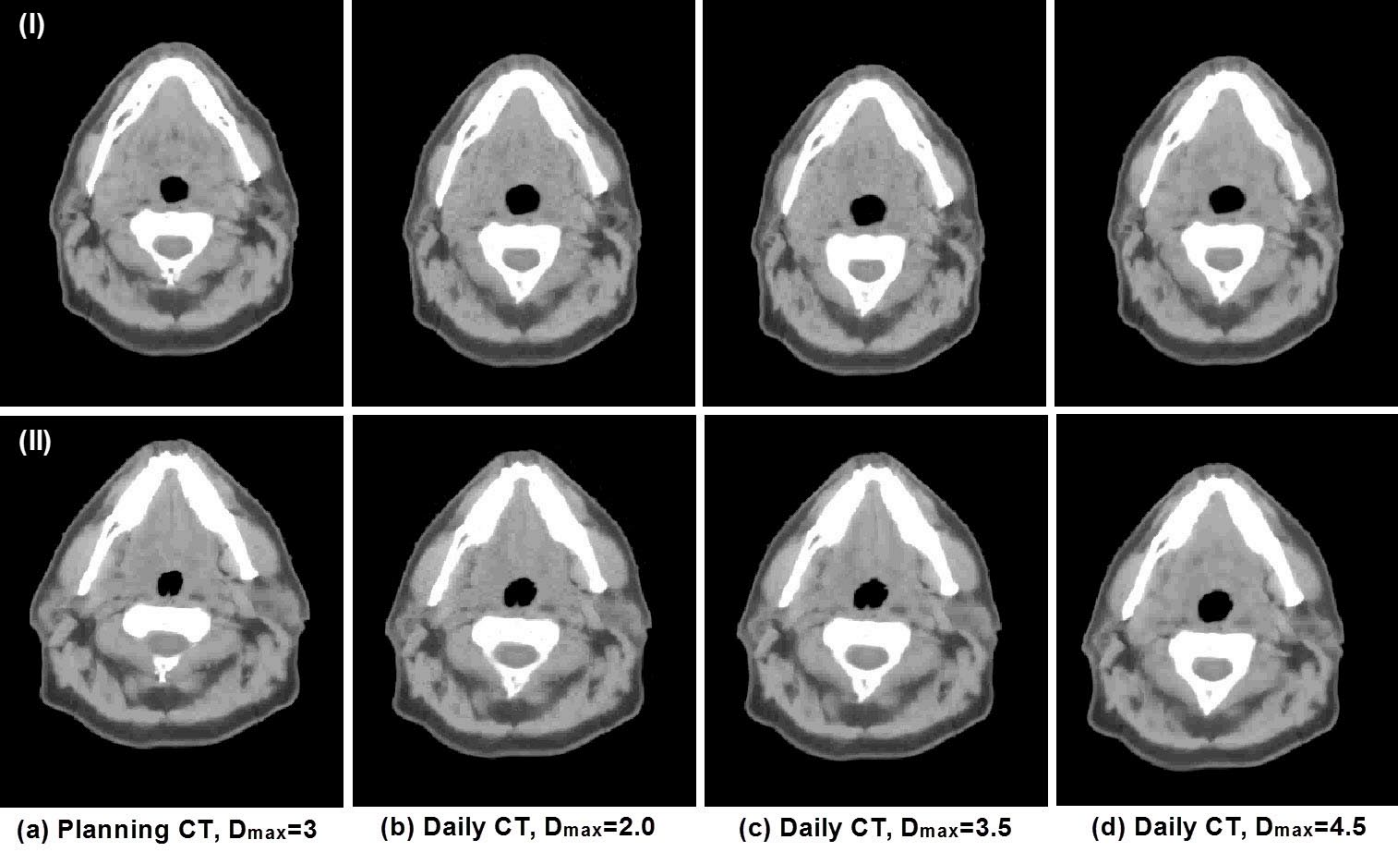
Two sets of artificial CT images generated from active shape models with each set in a row. The first column is the artificial planning CT, and the other three columns are artificial daily CT images with varied levels of variations specified by $D_{\text{max}}$.
